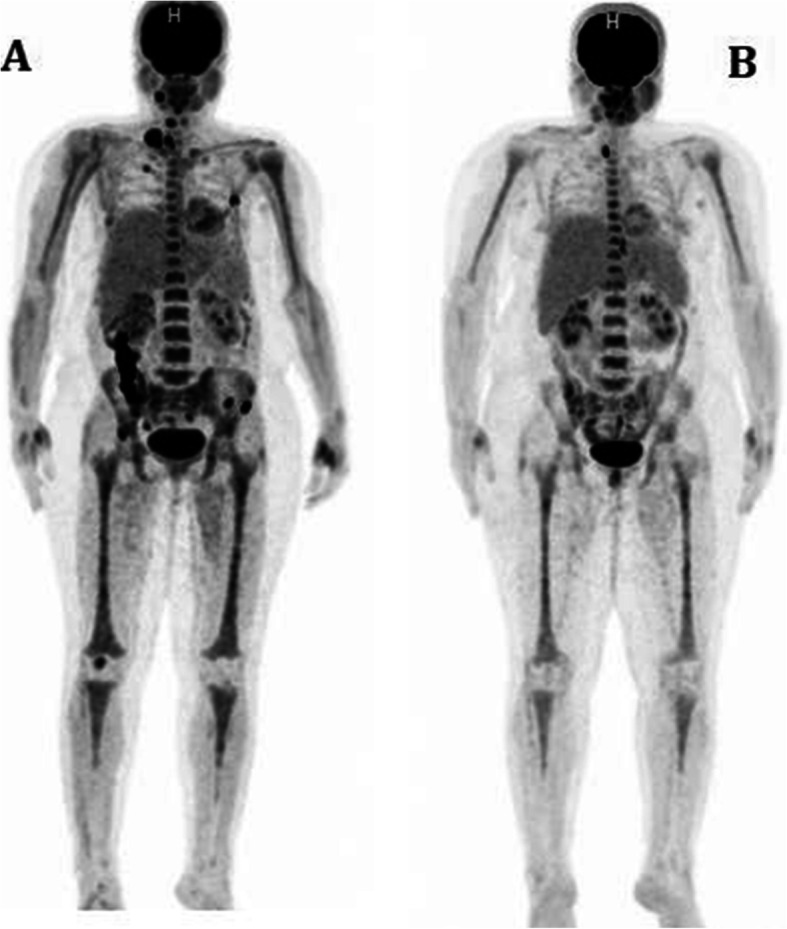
Pre- and postoperative maximum intensity projection images of ^18^F-FDG PET/CT: FDG-avid multiple skeletal lesions in the preoperative FDG PET/CT scan maximum intensity projection image (A) that shows complete metabolic resolution in the postoperative ^18^F-FDG PET/CT MIP image (B)
